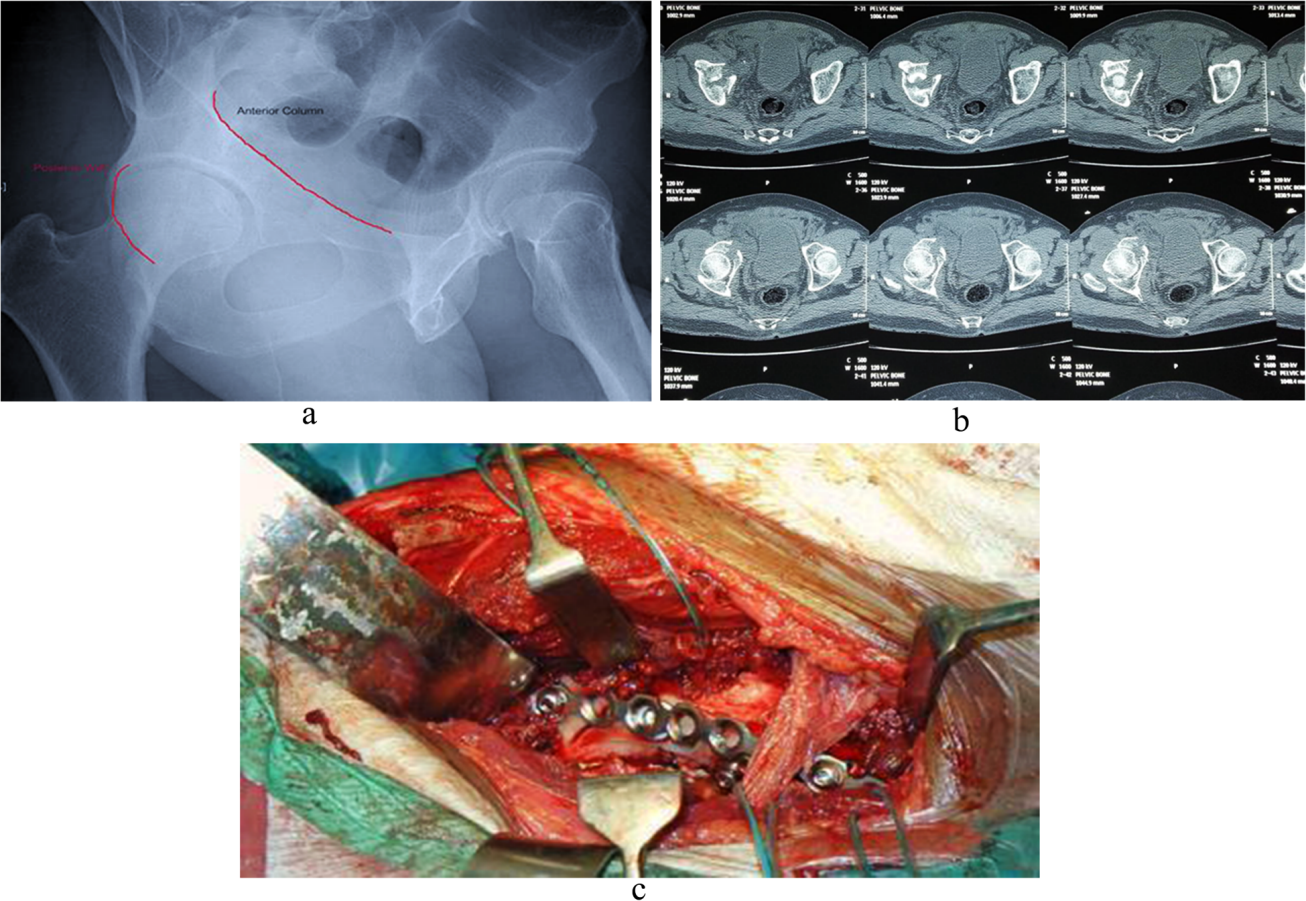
Anterior column fracture AP view (a) and axial CT preoperative (b) and intraoperative (c)
